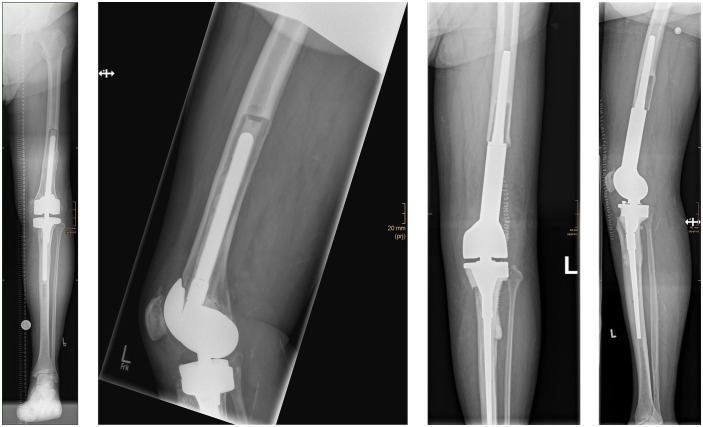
Example radiographs of an elective patient: (a) preoperative full-length anteroposterior view, (b) preoperative lateral view, (c) postoperative anteroposterior view, and (d) postoperative lateral view following distal femoral replacement.
